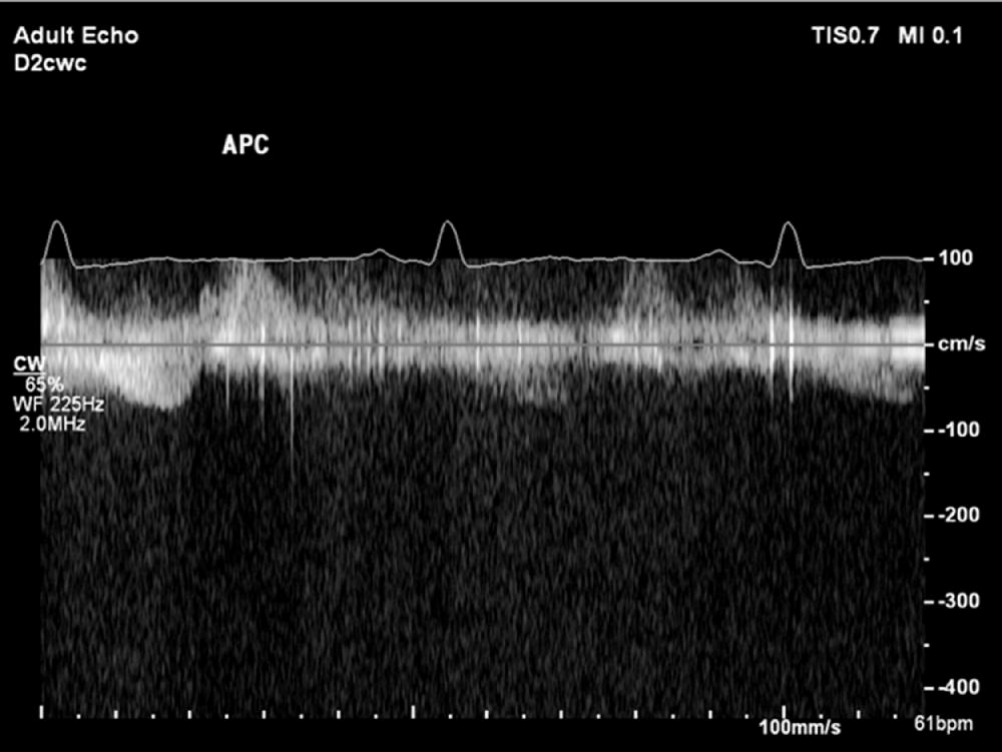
Repeat transthoracic echocardiogram using the non-imaging continuous wave Doppler probe from the cardiac apex shows poor continuous wave Doppler waveform.
